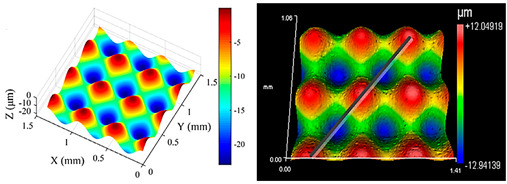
Liu et al. [10]	Radial ultrasonic vibration-assisted turning
